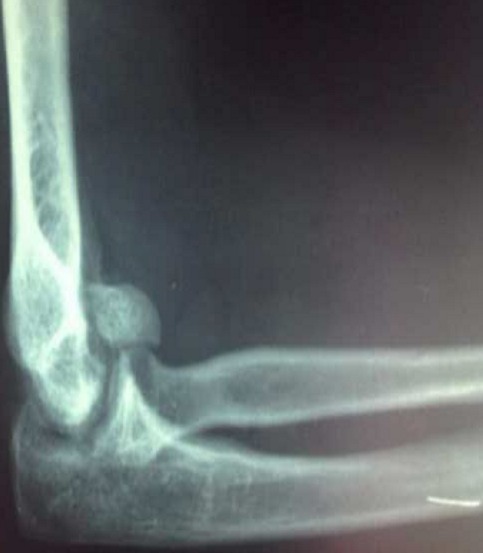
Radiographie du coude droit de profil montrant le cal vicieux du capitellum
